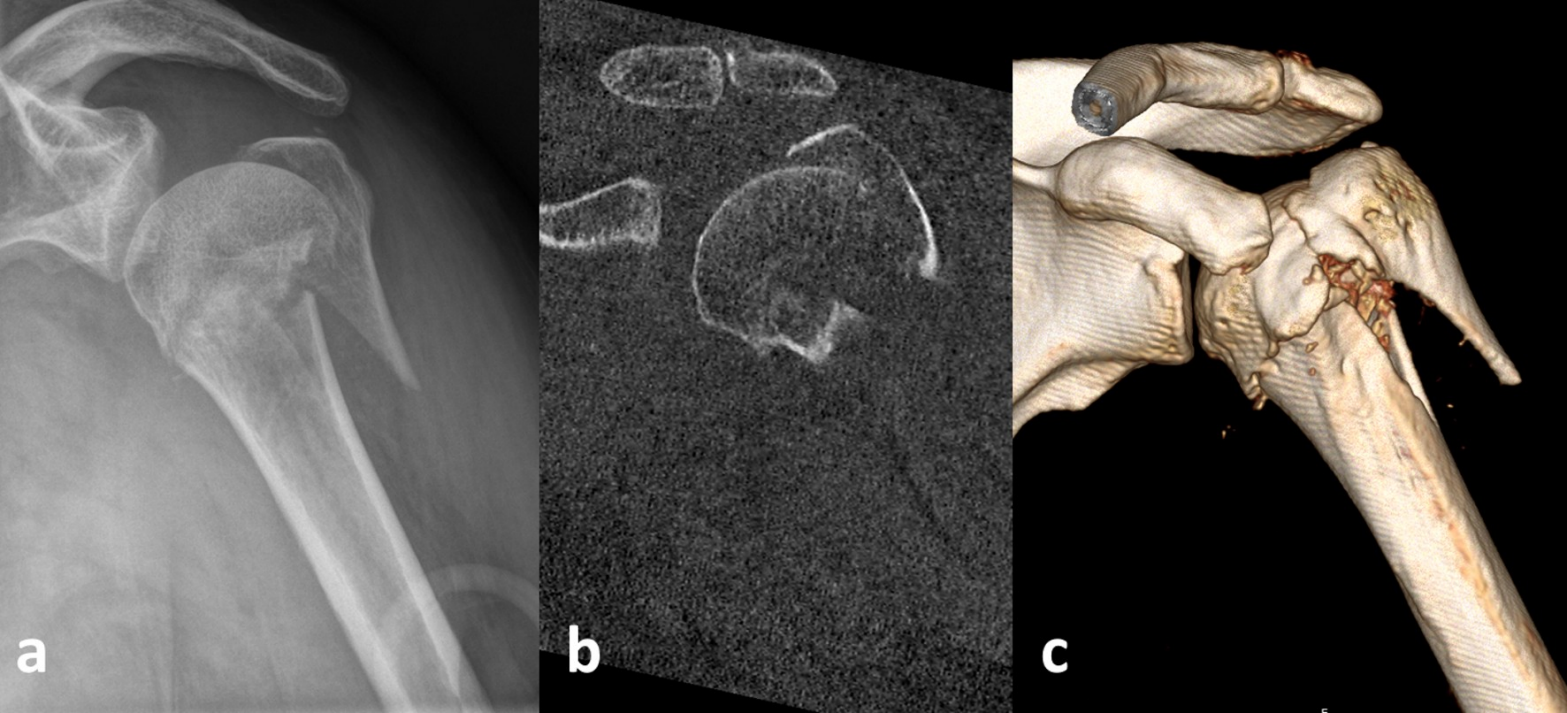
Split type 2 of GT fragment according to the morphological Mutch classification in a multi-part fracture of a 62-year-old patient. Plain radiograph (a), 2-D CT (b), and 3-D CT (c).
